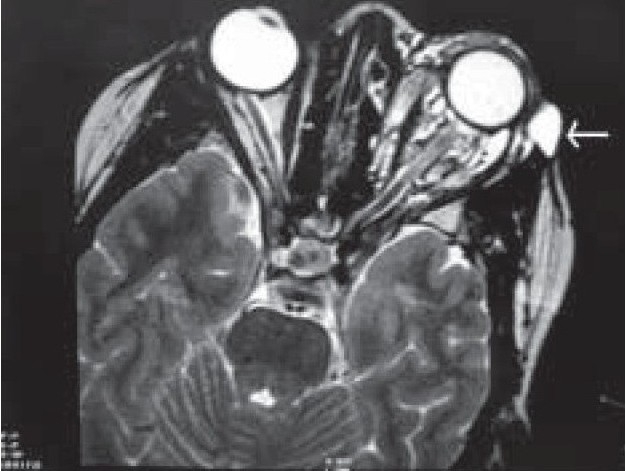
T2-axial MRI image shows extraconal extension (Arrow)
